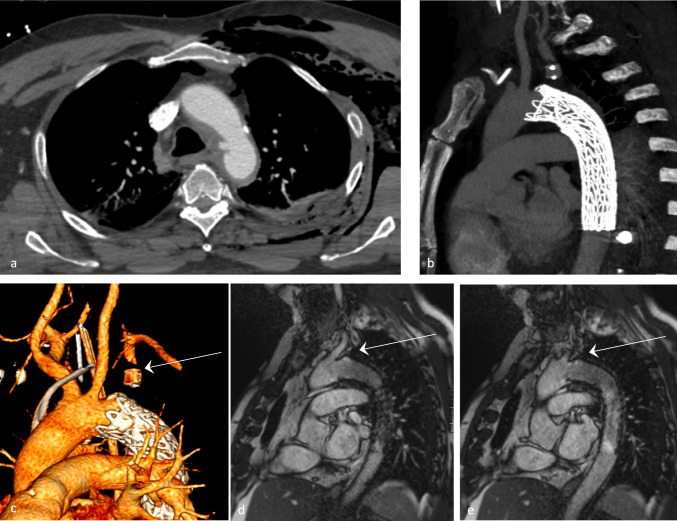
Grade III aortic isthmic lesion treated with Gore C-TAG 34 × 150 mm with coverage and exclusion of LSA (Amplatzer Vascular Plug 12 mm, arrow). a Preoperative CTA; b postoperative CTA and c volume rendering reconstruction; d e 31-months follow-up MRI: no device-related complications
